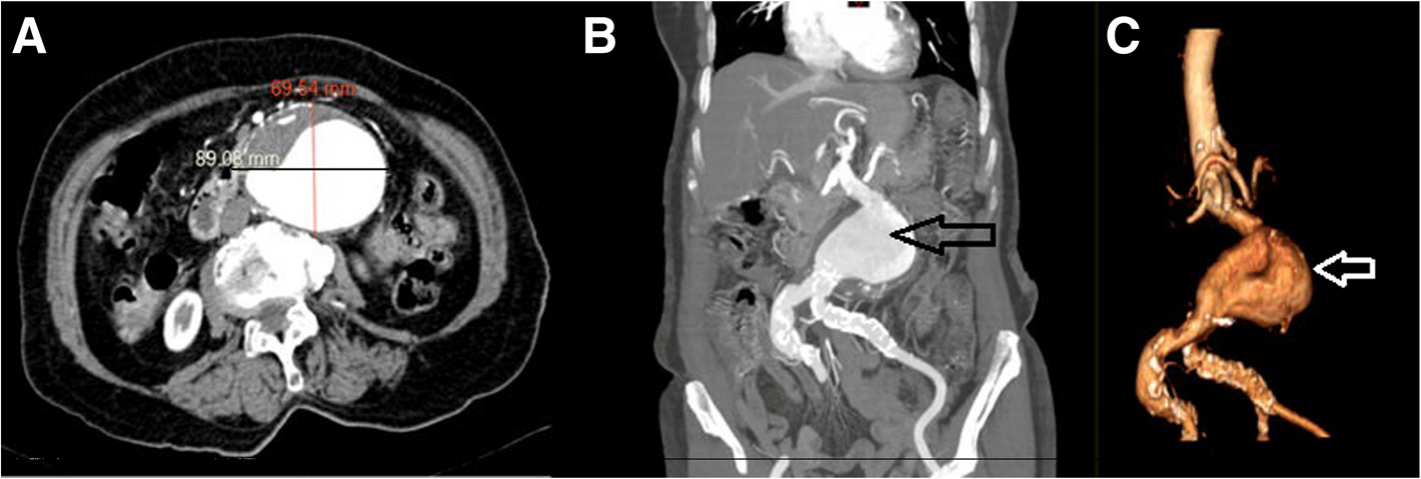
Contrast Tomography Angiography (CTA) in a 66 year old woman. Axial view (a) demonstrates a maximum diameter of 89 mm (mm) and antero-posterior diameter of 69 mm. Coronal View (b), and three dimensional (3D) reconstruction (c) black and white arrows indicate the aneurysm
